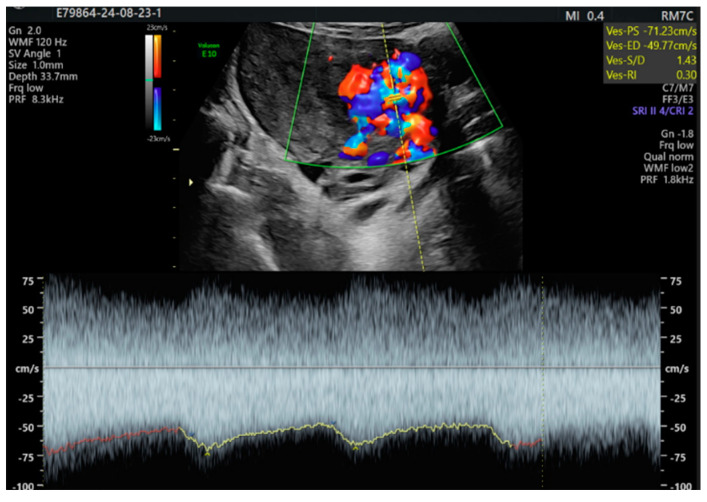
Pulsatile Doppler US image with turbulent flow: the spectral Doppler shows a flow with low resistance in the arterial vessel.
